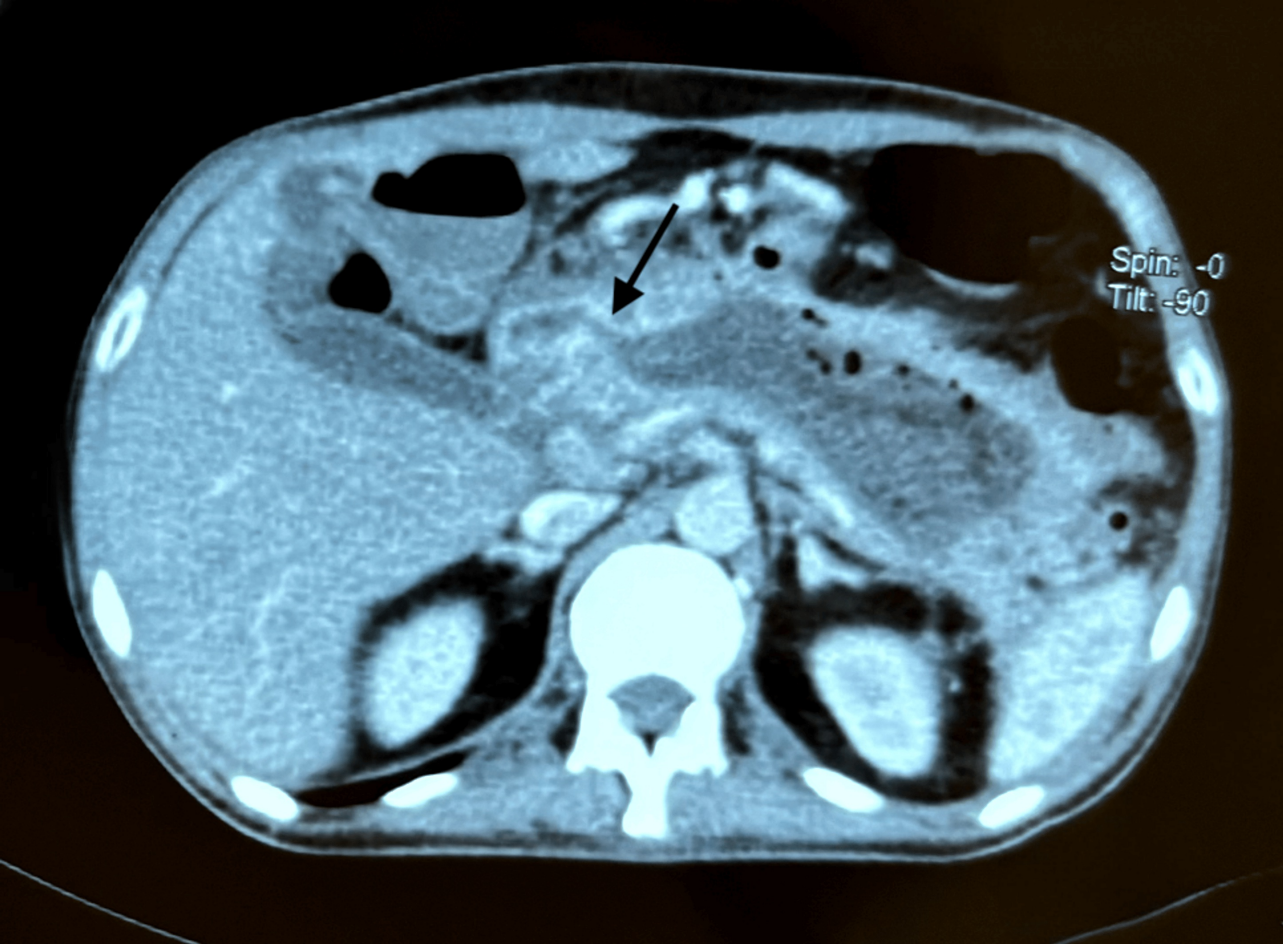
Abdominal CT scan in axial section showing an abnormal fistulous communication between a pancreatic pseudocyst and the first (D1) segment of the duodenum (arrow).
